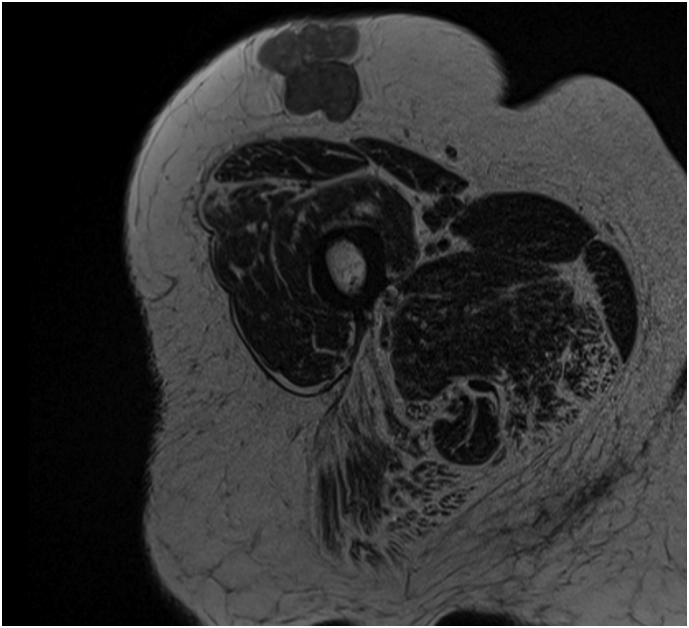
MRI image of the subcutaneous lesion.
